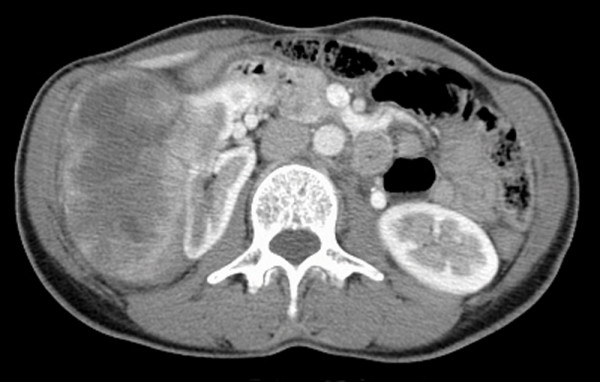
CT of the abdomen. Arterial phase images of dynamic computed topography scan showed a highly necrotic tumor compressing the renal parenchyma without either invasion to surrounding tissues or local lymphadenopathy.
